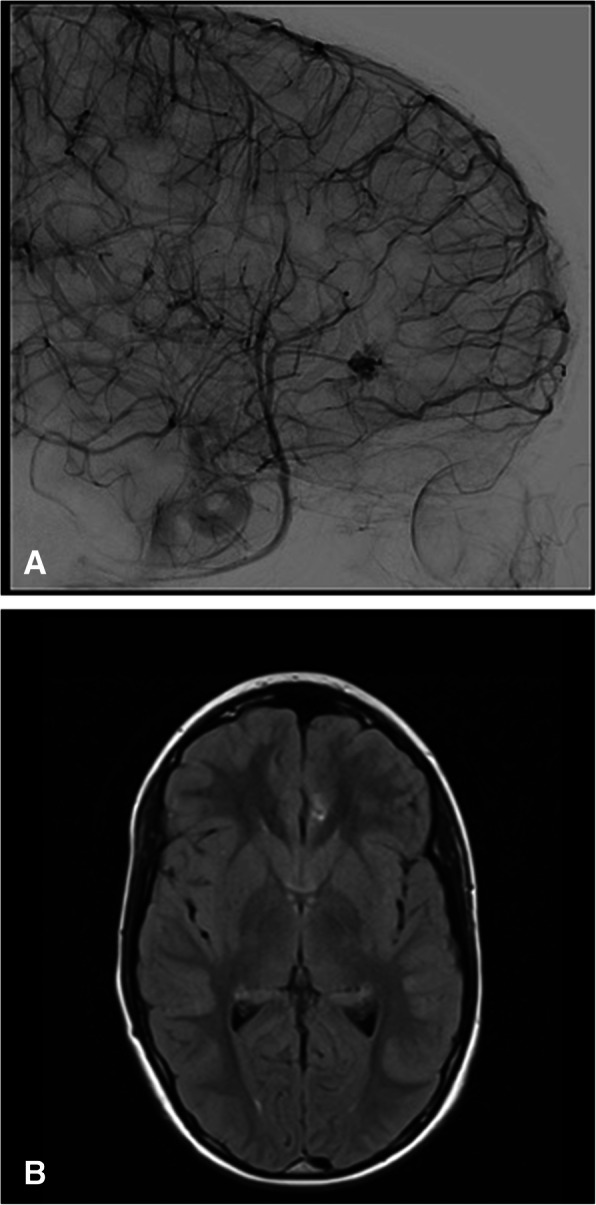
A and B 12-year-old boy with heterozygous ENG mutation hereditary hemorrhagic telangiectasia and a cerebral capillary vascular malformation. A Late arterial phase lateral projection digital subtraction angiogram of the left internal carotid artery demonstrates a sub centimeter focus of ectasia and blush without arteriovenous shunting in the medial aspect of the left frontal lobe (arrow). B Axial FLAIR sequence showing corresponding focus (arrow)
